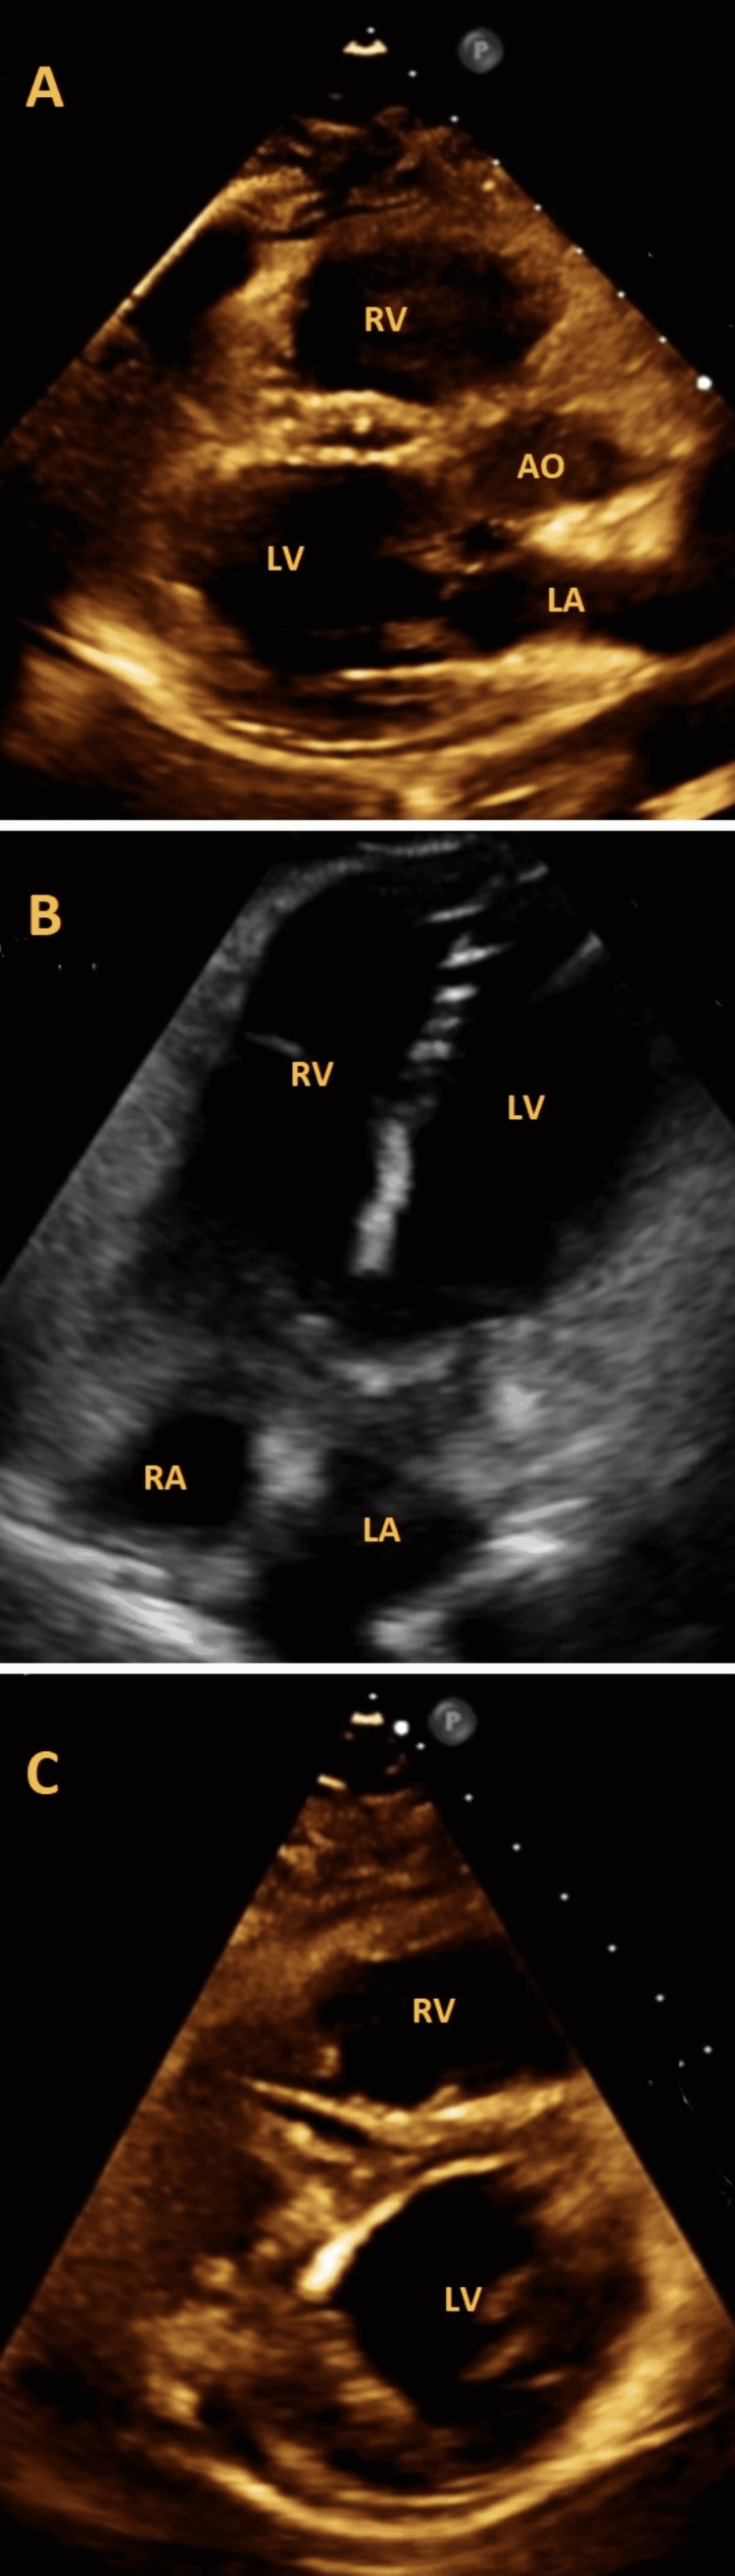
(A) Parasternal long axis, (B) apical four chamber view, and (C) parasternal short axis view showing a dilated right ventricle (RV) on transthoracic echocardiogram (TTE).
